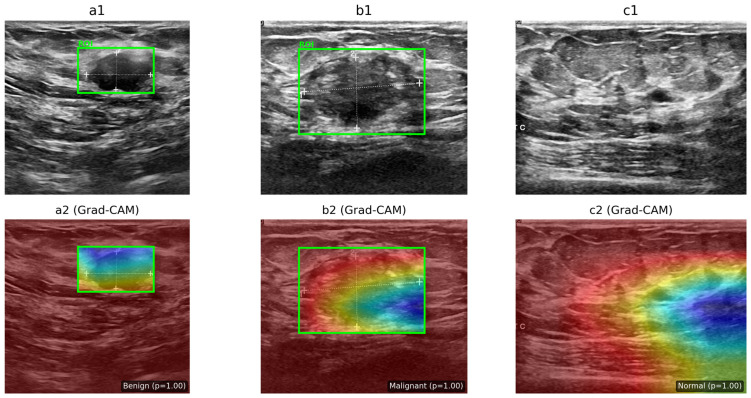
Interpretability analysis using Grad-CAM on BUSI ultrasound images. The upper row shows BUSI ultrasound samples for (a1,a2) benign, (b1,b2) malignant, and (c1,c2) normal cases with radiologist-defined regions of interest (ROI). The bottom row presents the corresponding Grad-CAM heatmaps generated by the EfficientNetV2 feature extractor. Benign and malignant cases exhibit localized activation around lesion boundaries, while the normal case shows diffused activation patterns, indicating the absence of abnormalities.
